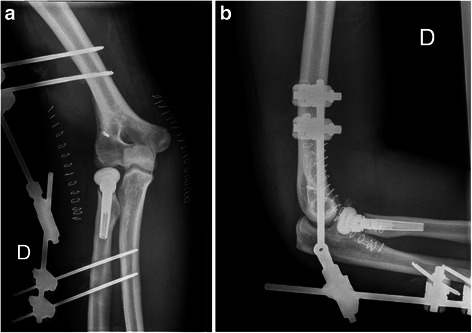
Postoperative radiographs: anteroposterior (a) and lateral (b) views. The radiographs show a radial head prosthesis *in situ*, indirect signs of collateral ligamentous repair (anchor), and a congruency of the ulnohumeral joint
